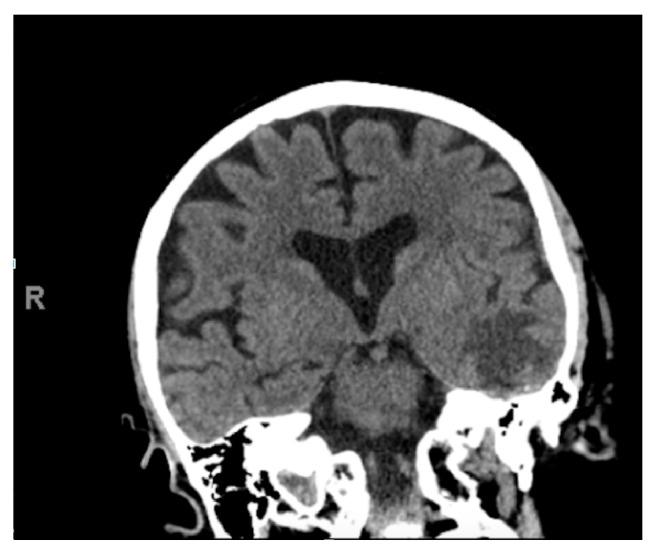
Coronal CT demonstrates hypodense left temporal lobe vasogenic edema.
